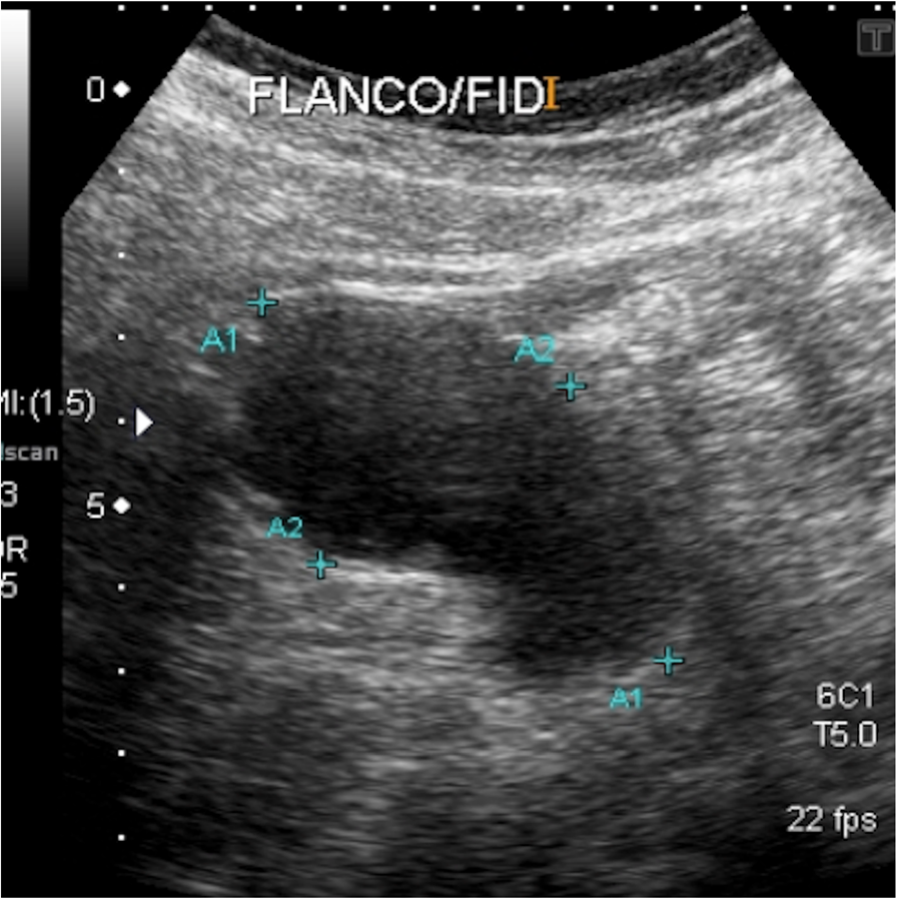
Dilated appendix, with a diameter of 3.5 cm compatible with appendiceal mucocele, in ultrasound
